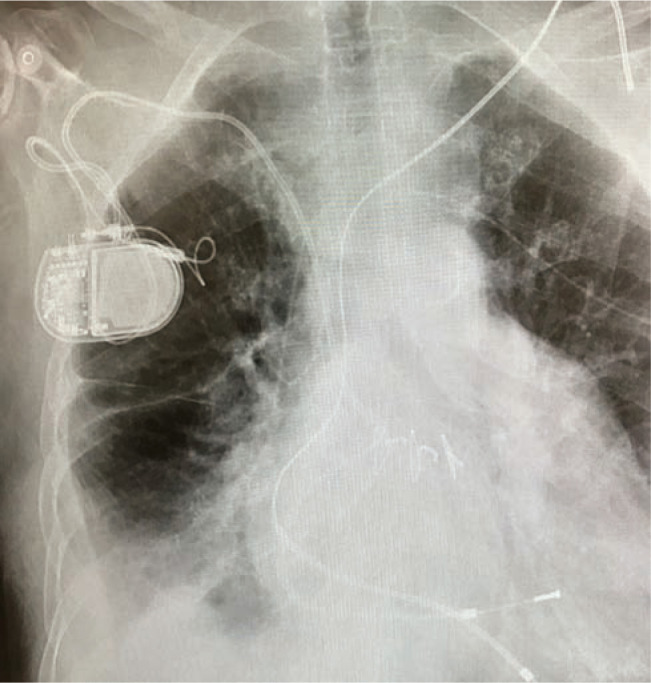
Preoperative chest X-ray reveals only two transve-nous electrodes despite 40 years of pacing therapy. Also noteworthy for marked cardiomegaly and a horizontal substernal approach.
